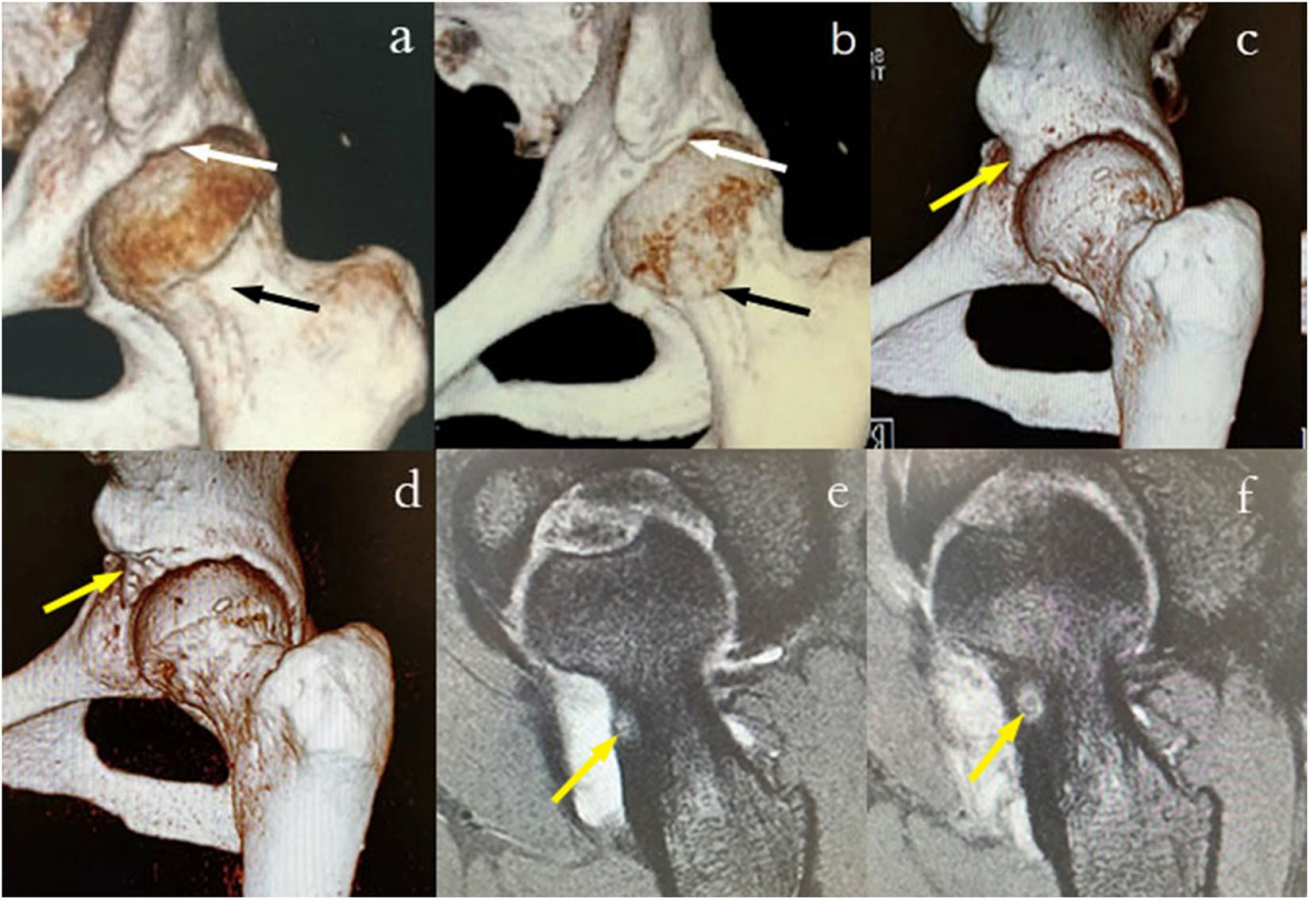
Preoperative and postoperative 3D CT and MRI. (a) Preoperative residual cam (black arrow) and pincer deformity (white arrow). (b) The outcome of femoral osteoplasty (black arrow) and acetabuloplasty (white arrow) after revision surgery (same patient as presented in (a). (c) Preoperative anterior inferior iliac spine/subspine impingement (yellow arrow). (d) The outcome of focal subspinal decompression (yellow arrow) after revision surgery (same patient as presented in (c). (e) Misdiagnosis of osteoid osteoma (yellow arrow) before the first arthroscopic surgery. (f) The image of osteoid osteoma (yellow arrow) before revision surgery (same patient as presented in (e).
